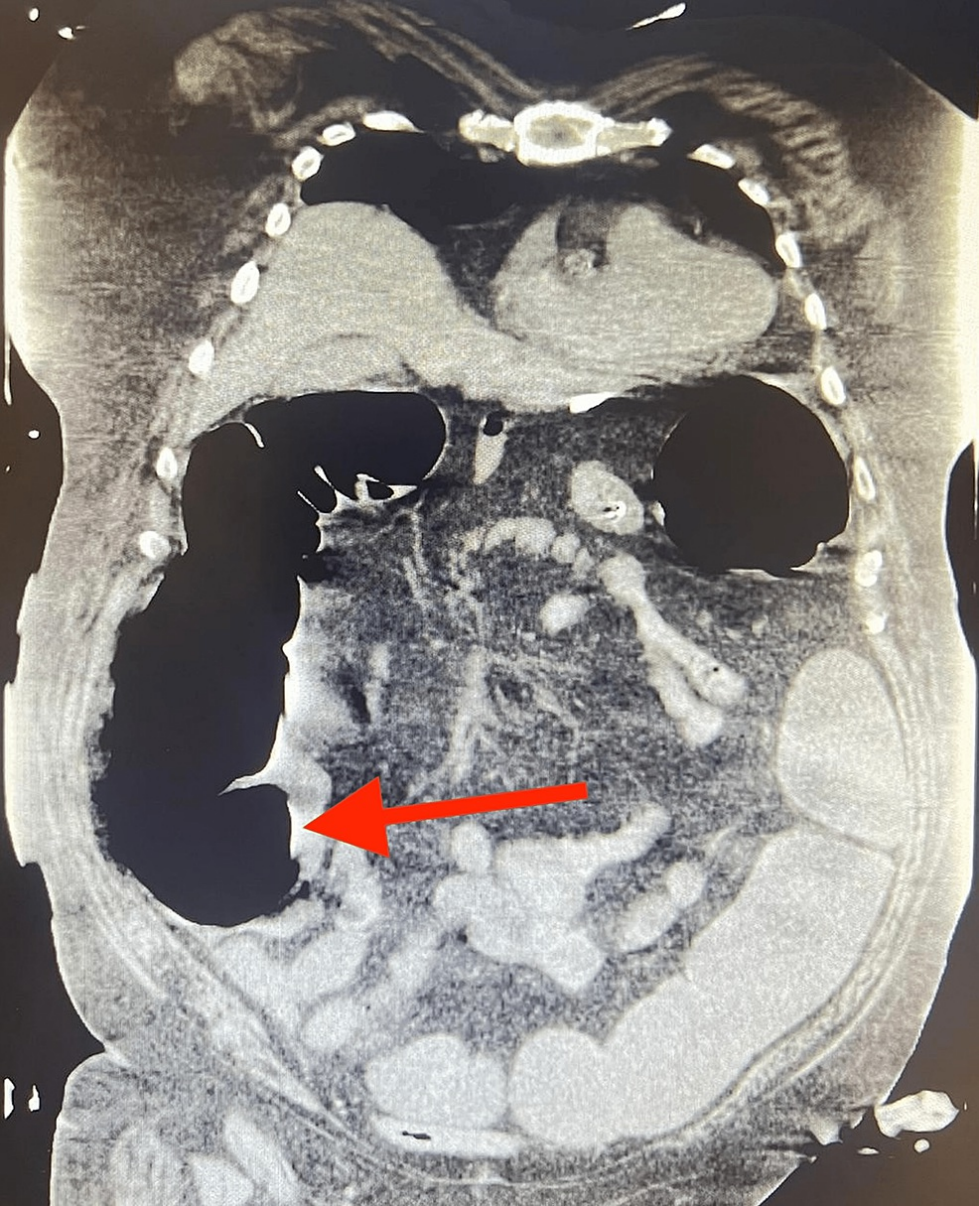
CT of the abdomen and pelvis without contrast showing dilated large bowel segments without transition point or obstructing lesion
